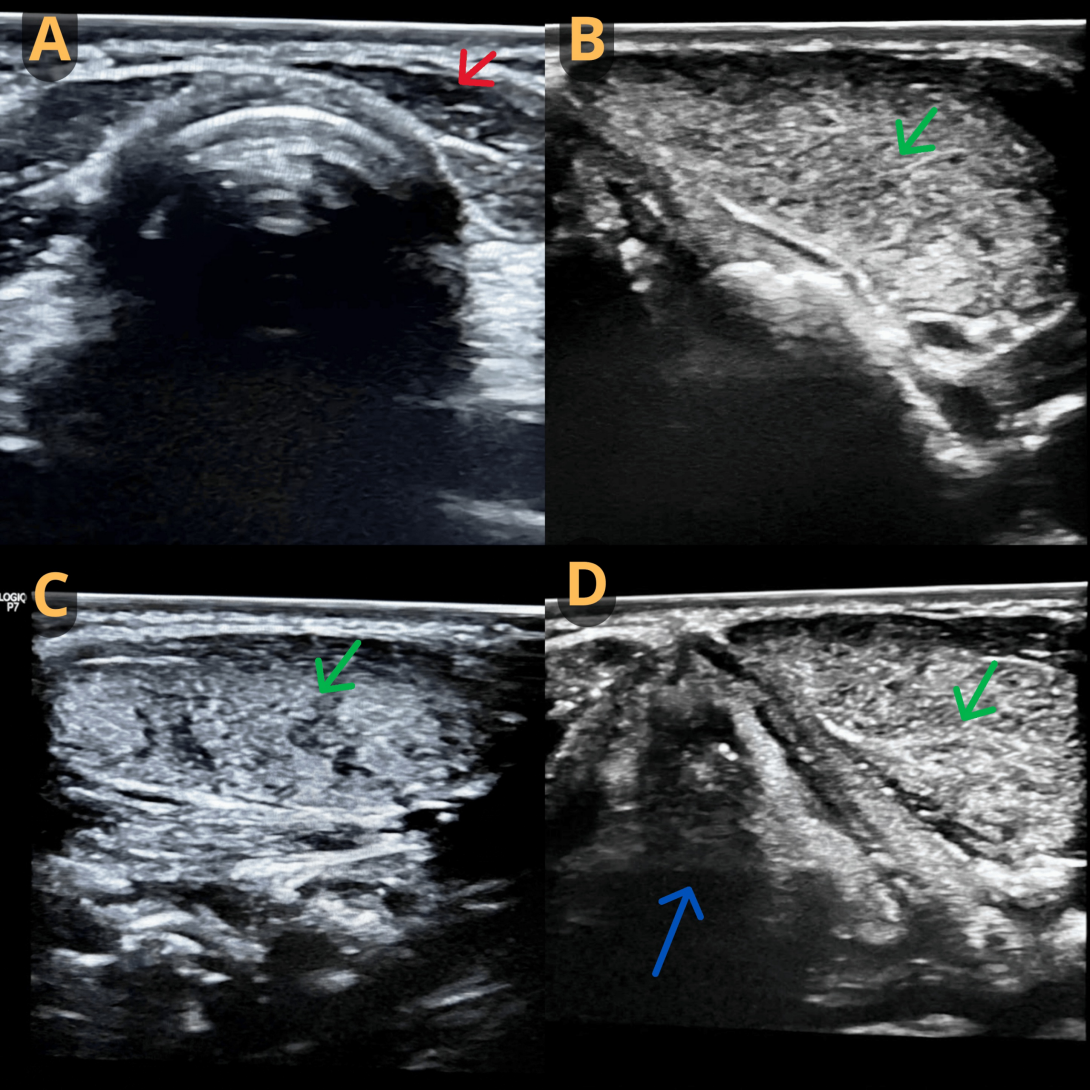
Ultrasonography of the cervical region Absence of an orthotopic thyroid gland (A), hyperechoic nodule in the right paramedian anterior cervical region (B) adjacent to the laryngeal thyroid cartilage (C, D). Red arrow: empty thyroid gland; Green arrow: thyroid parenchyma; Blue arrow: larynx
